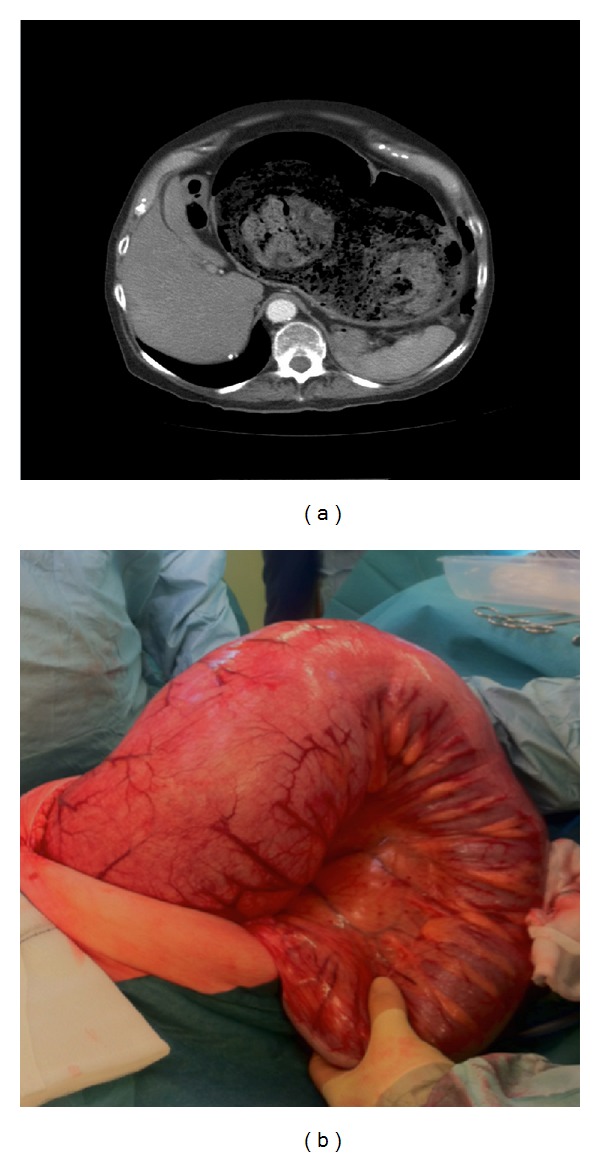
(a) CT scan showing grossly distended sigmoid containing faeces. (b) Sigmoid colon at laparotomy.
